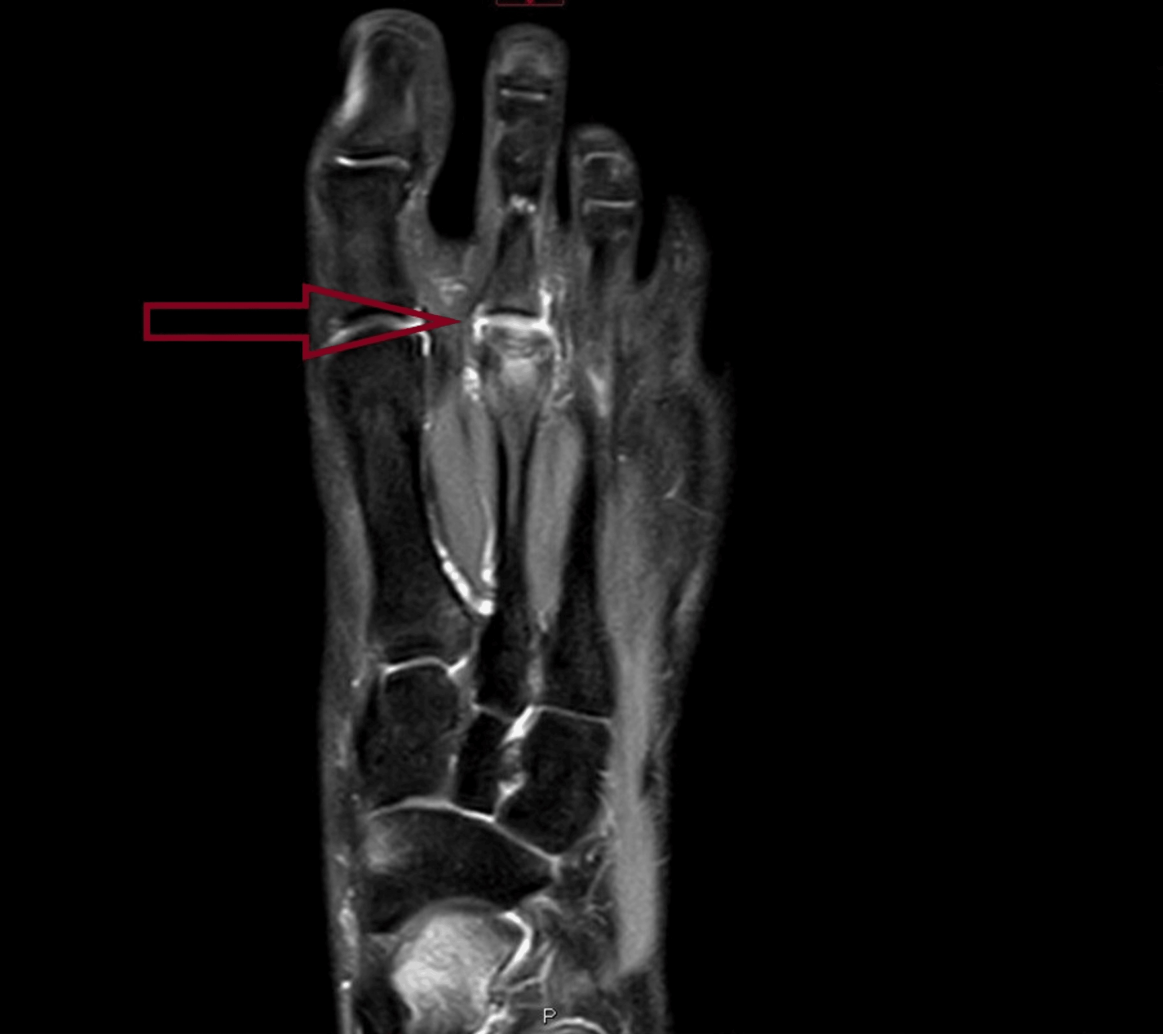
MRI showing collapse of the second metatarsal head with surrounding edema.
